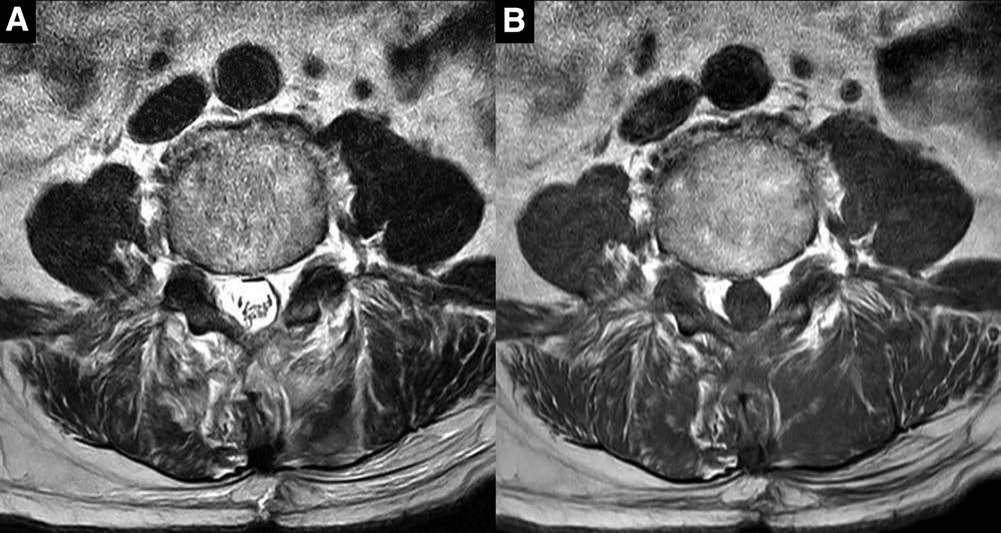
Axial (A) T2-weighted and (B) T1-weighted images taken at 5 days after the decompressive laminectomy showing well-decompressed spinal canal at the L4 to 5 level and no newly developed pathologies.
